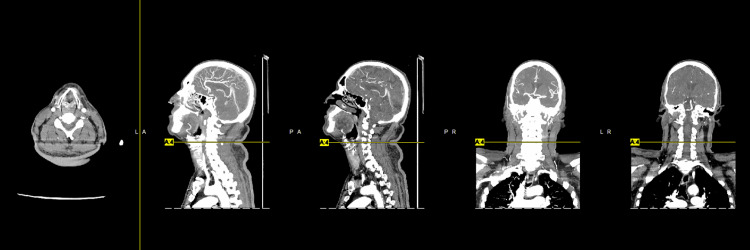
CT angiography of the neck demonstrating no hemodynamically significant carotid artery stenosis.
